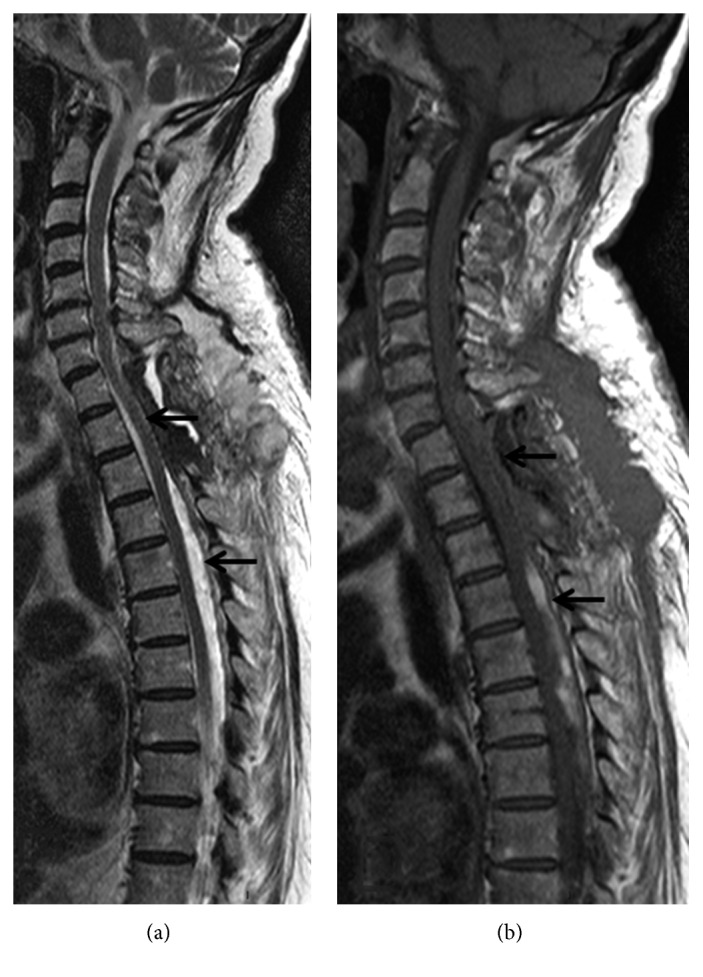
Postsurgical MRI scan. Sagittal views: T2 (a) and T1 (b). Resolution of spinal cord compression in the operated segment (D1–D3) upper arrows, with persistence of cord compression at the D4–D7 level (lower arrows).
